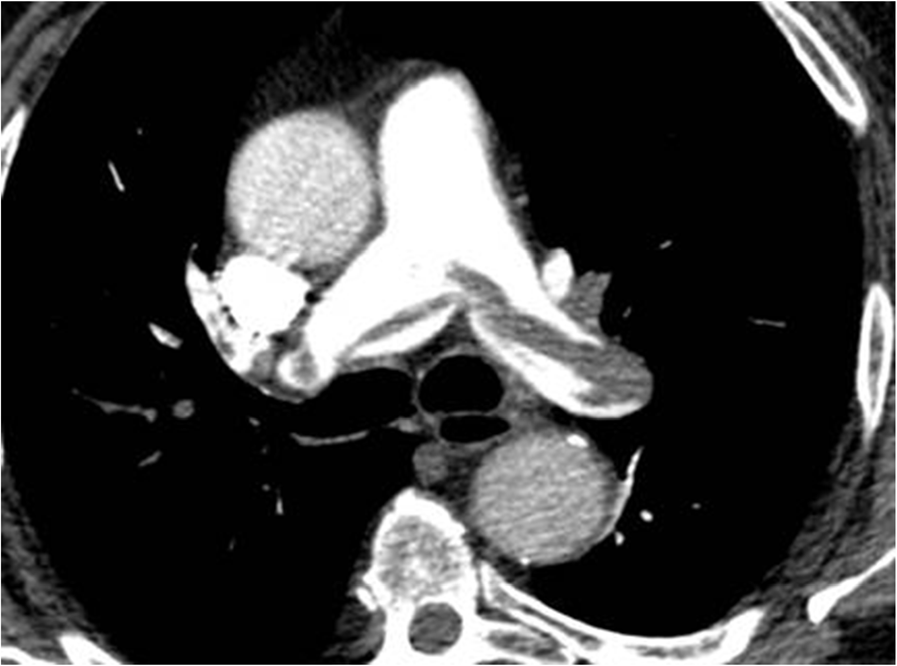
Proximal bilateral pulmonary embolism on computed tomography scan in a mechanically ventilated ICU patient
